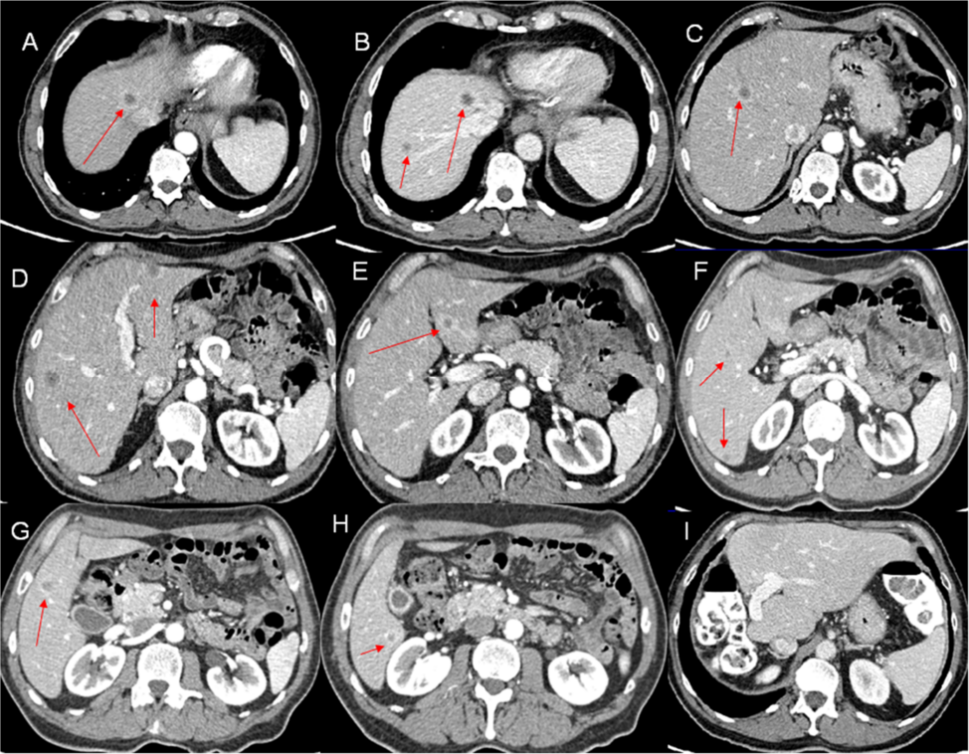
Computed tomography scan of liver metastases prior and after surgery: axial view A– H). Liver metastases in all liver segments except segment 1. I) CT scan after surgery. extended right hepatectomy showing no recurrence.
